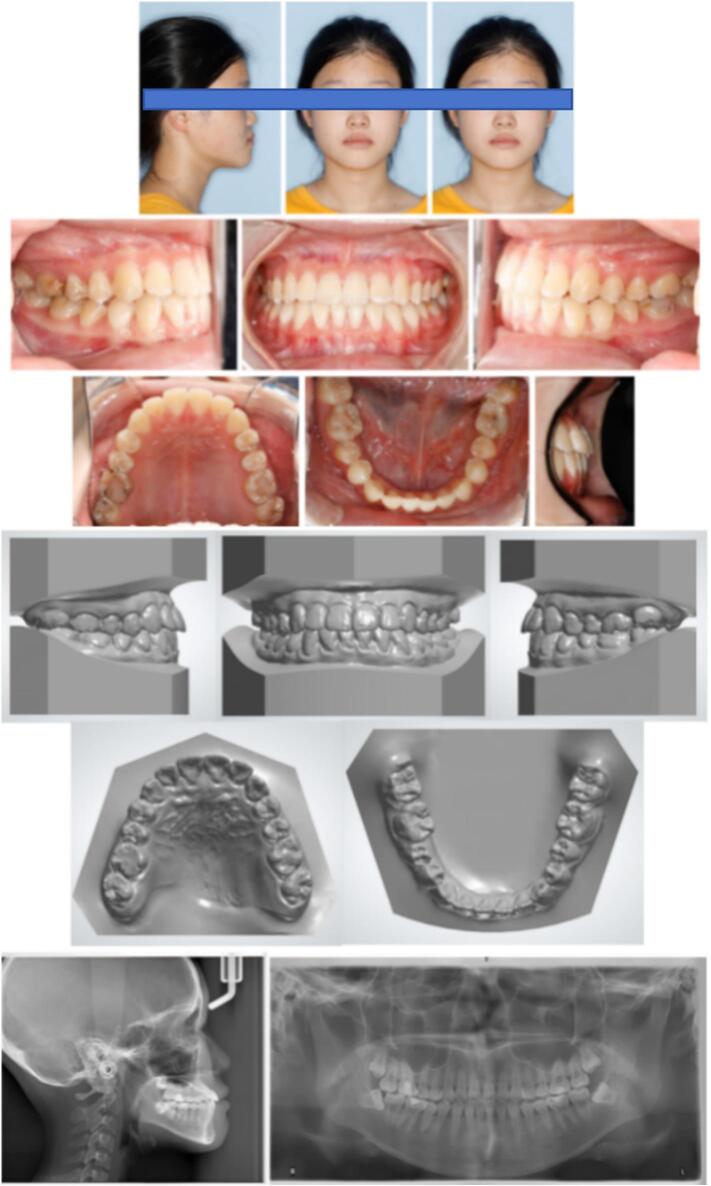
Post-treatment facial and intraoral photographs, post-treatment dental casts and post-treatment radiographs.
